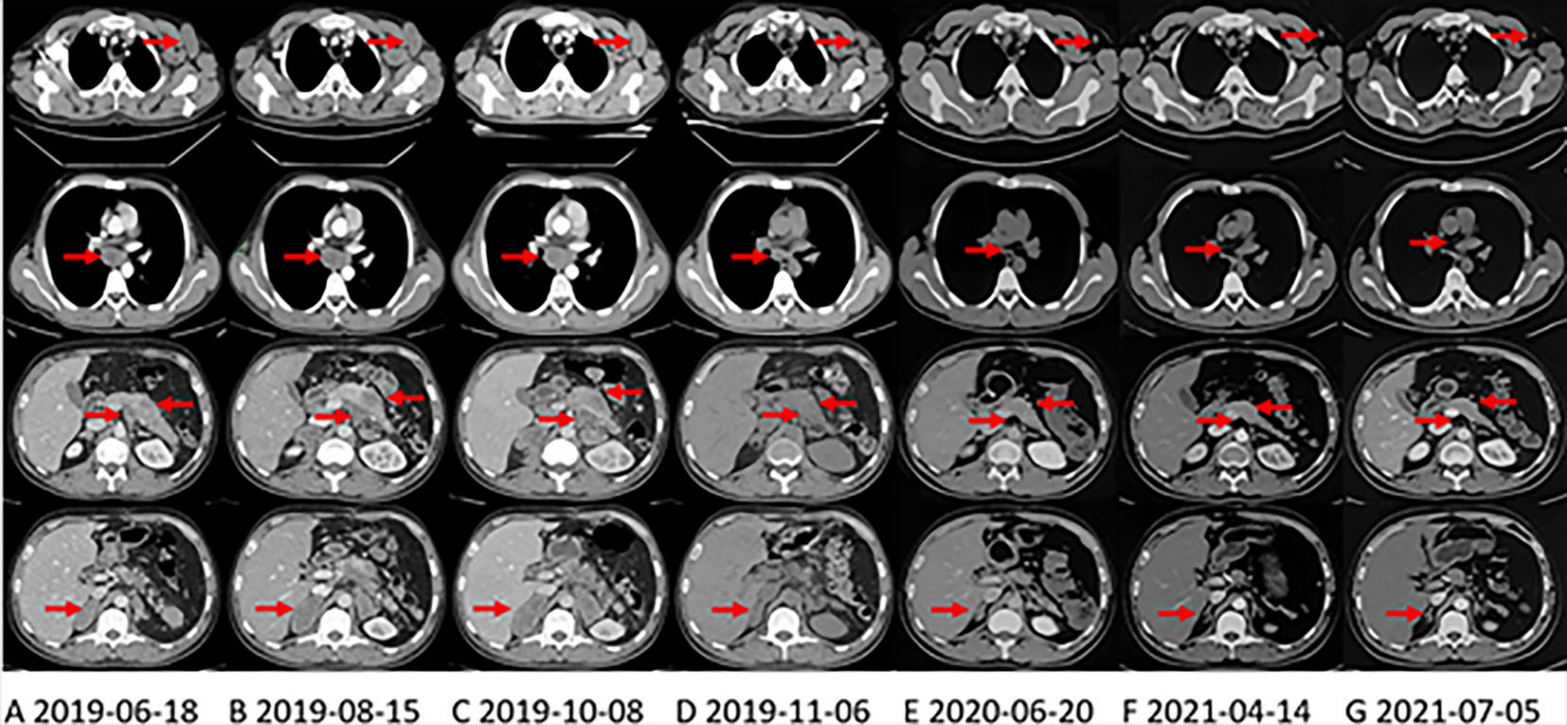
CT shows the changes of primary pancreatic lesion and metastases in the right axillary, mediastinum, and left adrenal gland with treatment. The arrows in each layer from top to bottom refer to the left axillary lymph node, mediastinal lymph node, pancreas and abdominal lymph nodes, and the right adrenal gland. (A) CT at the first evaluation of the patient. (B) CT evaluation of progressive disease (PD) after first-line irinotecan, oxaliplatin and capecitabine (XELOXIRI) treatment for 3 cycles. (C) CT evaluation of PD after second-line paclitaxel and gemcitabine treatment for 2 cycles. (D) third-line Crizotinib treatment One month later, the patient’s tumor shrank significantly, and CT was evaluated as partial response (PR). (E) when the patient was initially found to have brain metastases, the extracranial lesions were still stable, and the patient continued to take Crizotinib. (F) On April 13, 2021, The extracranial lesions are stable and the patient has been receiving Alectinib since June 2020. (G) at the latest CT evaluation, the extracranial lesions are still stable.
